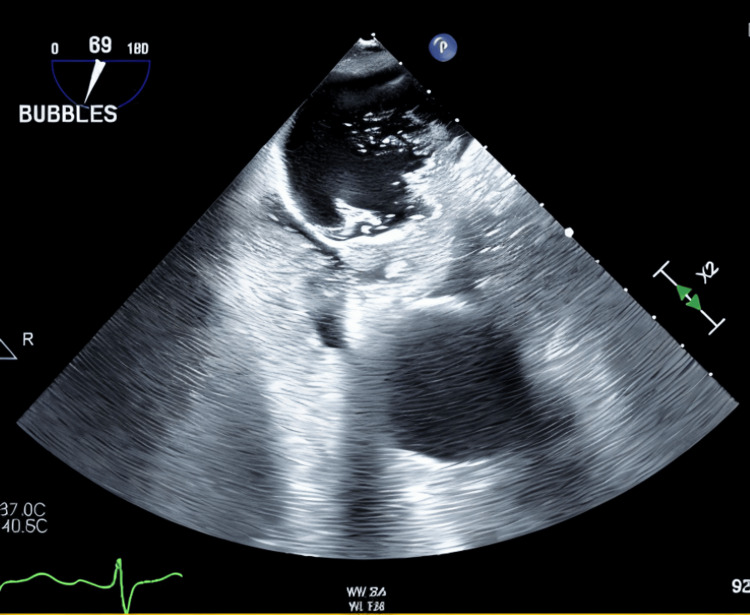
TEE 2D view with bubble study Still frame from agitated saline-enhanced TEE image demonstrating dense opacification of the right atrium with agitated saline contrast. Then microbubbles enter the left atrium almost simultaneously, within one cardiac cycle of right atrial opacification. This is consistent with a right-to-left intracardiac shunt. Findings consistent with an ASD. ASD: atrial septal defect; TEE: transesophageal echocardiography
